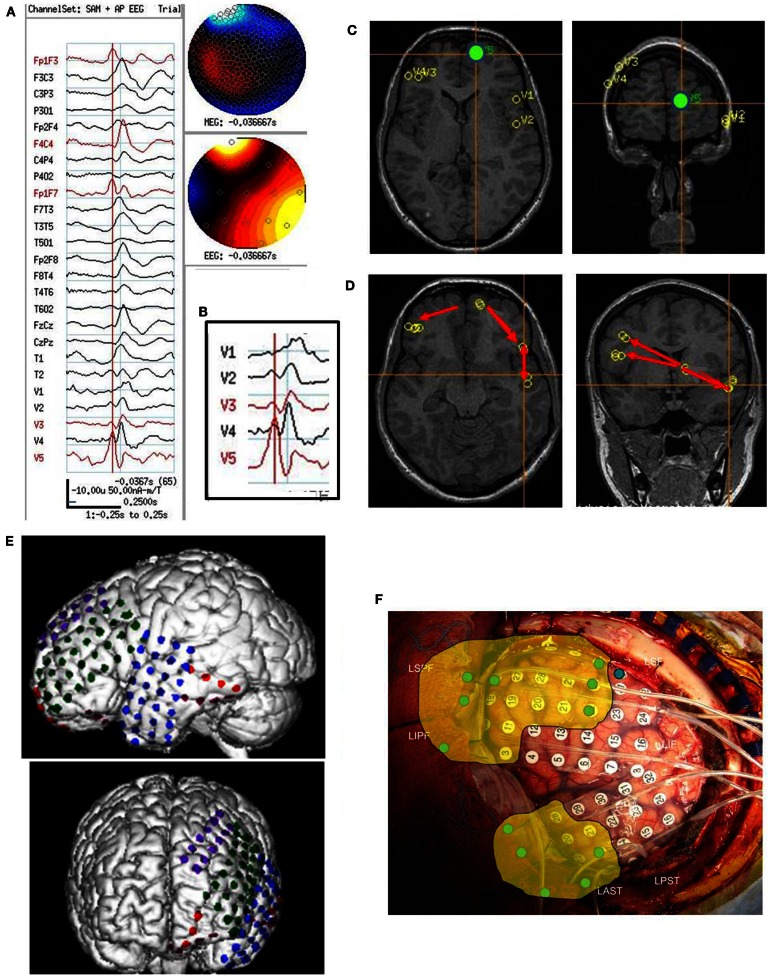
Subject 1. (A) EEG in bipolar AP montage and five virtual sensor channels showing average of 146 interictal spike epochs in bandwidth 3–70 Hz, digitized at 300 Hz. Topographic maps for MEG and EEG signals are top of head views centered at vertex Cz with anterior (nose) at top of map. For this averaged spike, epochs were aligned based on spike peak detected at VS location V3 in right frontal lobe in the 20–70 Hz bandwidth. (B) Inset is enlarged view virtual sensor (VS) channels showing timing of peaks. V5 peak occurs earliest followed by V4 (40 ms), V3 and V2 (50 ms), and V1 (63 ms). Note that although largest amplitude peaks at V1–V4 are delayed after V5 peak, each has a small peak at about the time of V5 peak. Onset of spike peak at V5 location precedes apparent onset at the other four VS locations. Although the averaged epochs shown were aligned based on the spike at V3, the earlier onset of a spike at V5 was detected. (C) Subjects MRI scan showing VS locations of V1–V5. Green dot is location of V5, earliest of the VS peaks. (D) Subject’s MRI scan showing one possible pattern of spread based on timing of peaks and shortest distances between VS locations. The arrows are only to show relative timing, since propagation and pathways cannot be discerned solely with spike peaks separated in time. (E) 3D reconstruction of subject’s MRI and subdural electrode locations. (F) Intraoperative photograph of left brain surface. Green dots represent electrodes showing spikes at onset of seizures. Ictal onset was in left frontal lobe at LIPF2–4 (red frontopolar electrodes and purple anterior frontal lobe electrodes) with rapid spread to left anterior temporal lobe (blue electrodes). Yellow overlay represents resection plan.
